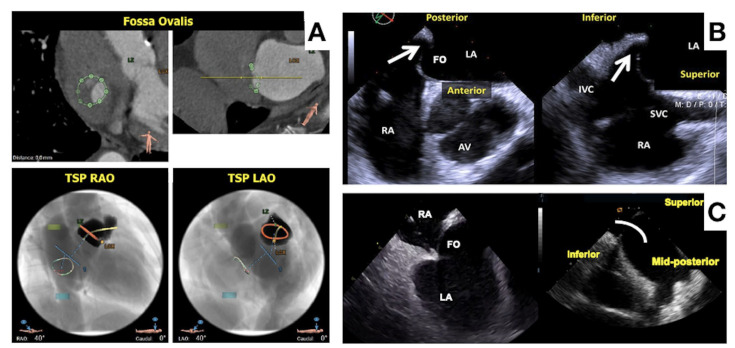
(A): CT planning for optimized transeptal puncture in superoinferior and antero-posterior planes; (B): transeptal puncture with TEE imaging; (C): transeptal puncture with ICE imaging.
